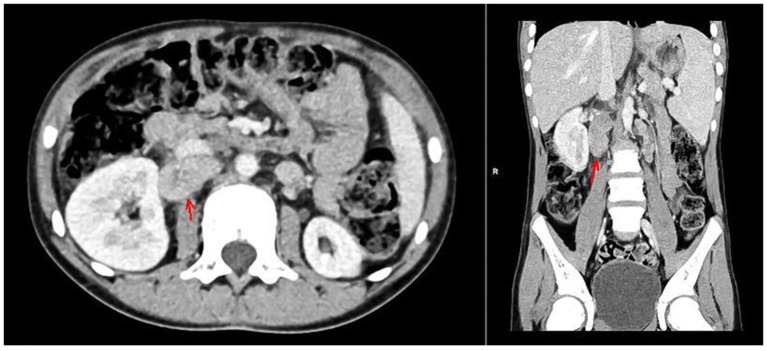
CT of the abdomen with contrast medium, arterial phase, axial, and coronal views. Hypodense foci in the II and VIII segments of the liver, enlarged spleen, *ca*. 14 cm long with nonhomogenous attenuation, hypoplastic left kidney, lymphadenopathy of lymph nodes in the regions of the head of the pancreas, portal vein, right renal hilum, inferior vena cava (marked with arrows), and retroperitoneal, iliac and aortic lymph nodes, forming a mass 8.5 cm long.
